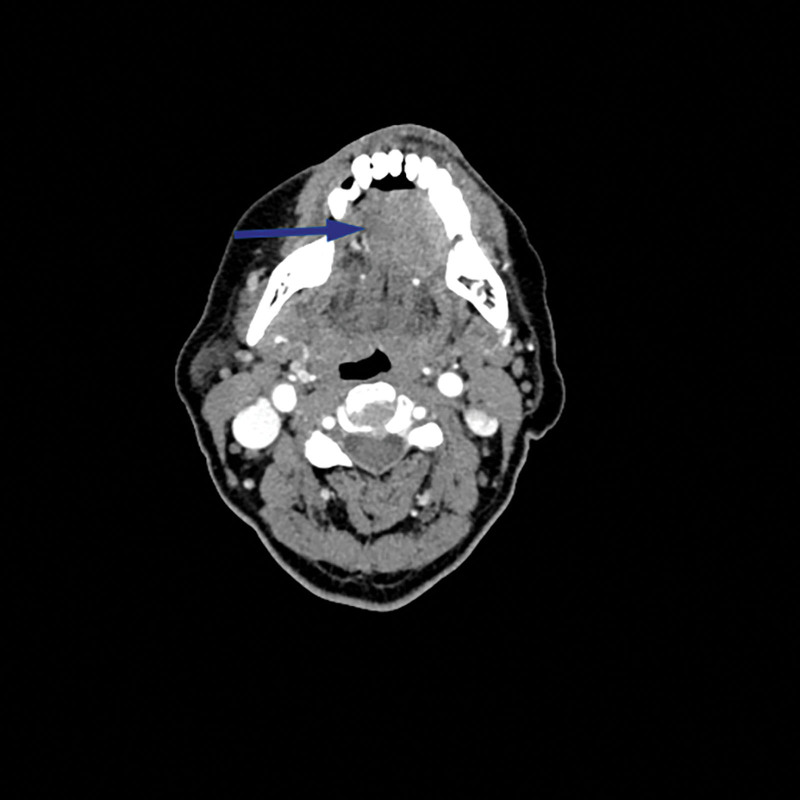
CT enhancement of the neck showing a left sublingual mass (blue arrow).
